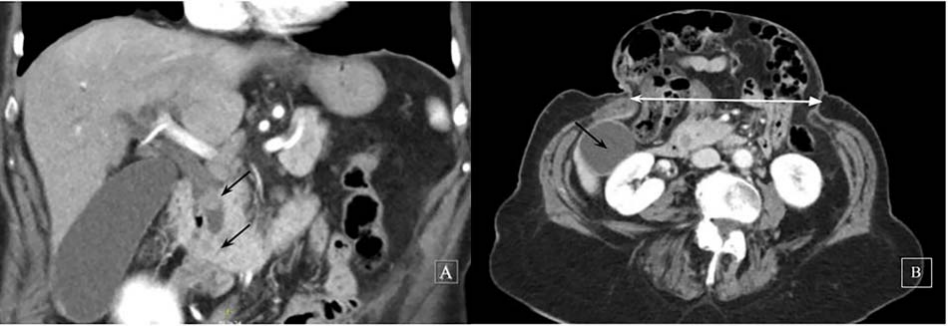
Contrast-enhanced computed tomography: a coronal image revealing two iso-attenuating filling defects in the distal bile duct causing obstruction (black arrows) (A) and a subcostal axial slice of a giant incisional hernia (horizontal distance 14 cm, white line, GB, black arrow) (B)
